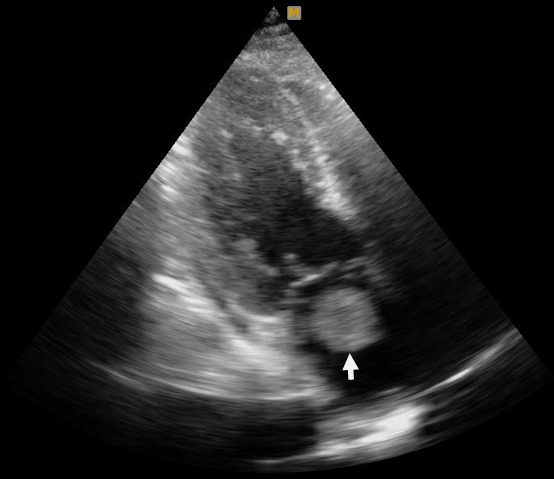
Echocardiography: left atrium myxoma
